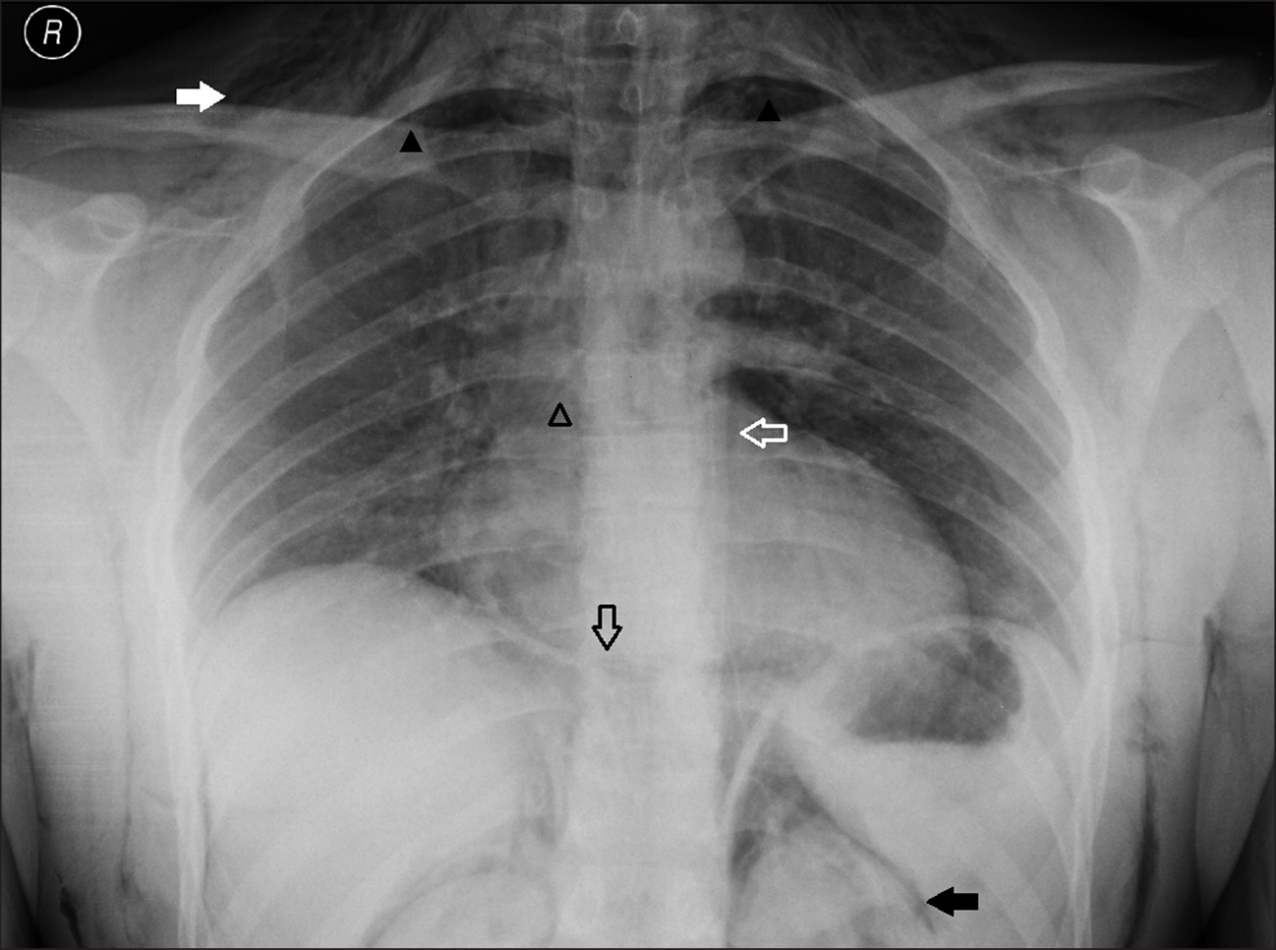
Anteroposterior (AP) chest radiograph demonstrating diffuse subcutaneous emphysema (solid white arrow), a small pneumothorax in both apical lung fields (solid black arrowheads) and air outlining the thoracic aorta (hollow white arrow), the bronchial branches (hollow black arrowhead), both sides of the diaphragm (hollow black arrow) and both kidneys (solid black arrow).
